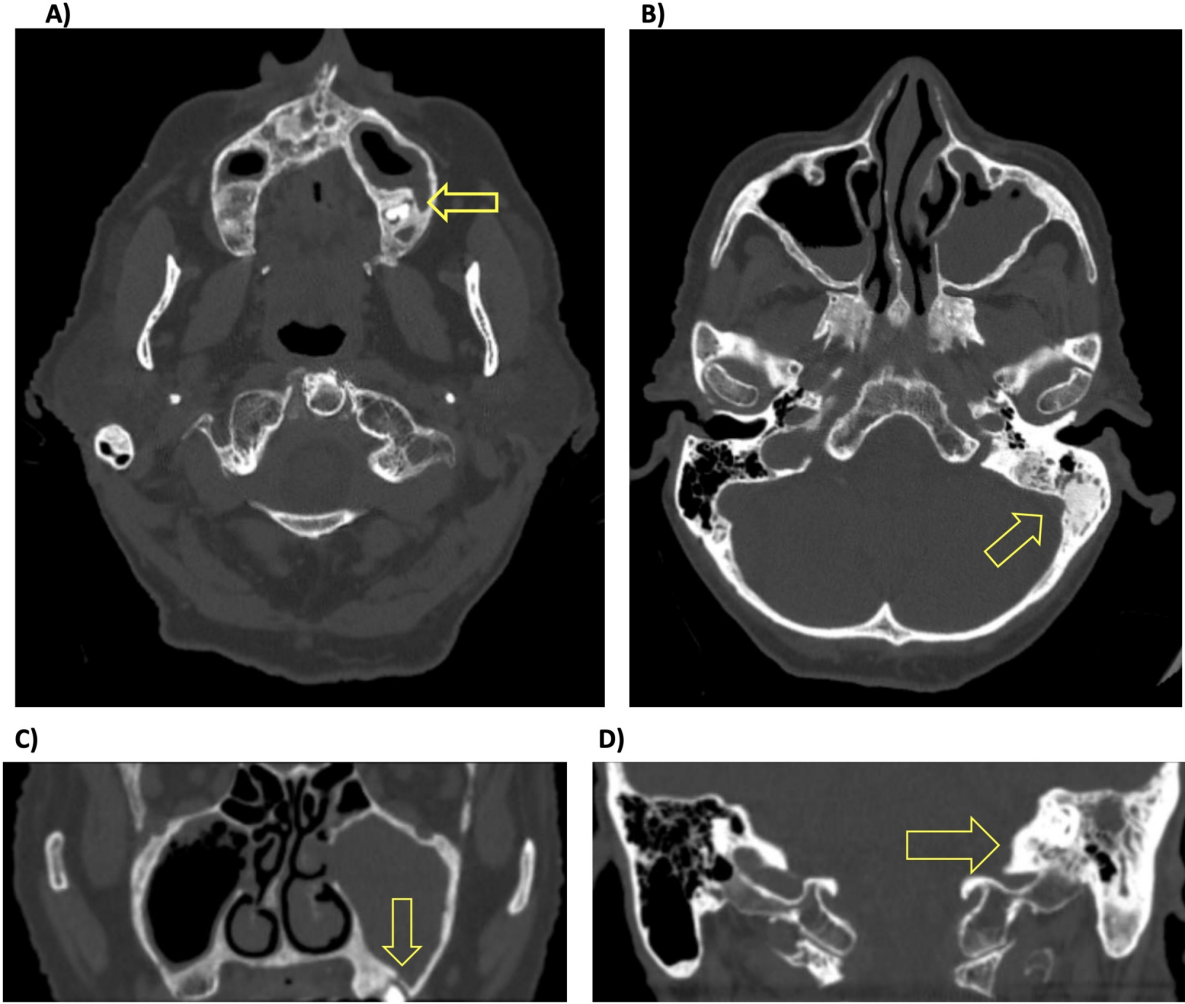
Computed tomography image of communication between periapical abscess and maxillary sinus, with ipsilateral maxillary sinusitis and mastoiditis. (A) Axillary view showing communication between molar abscess and maxillary sinus. (B) Axillary view demonstrating ipsilateral maxillary sinusitis and mastoiditis. (C) Coronal view of communication. (D) Coronal view of ipsilateral mastoiditis.
